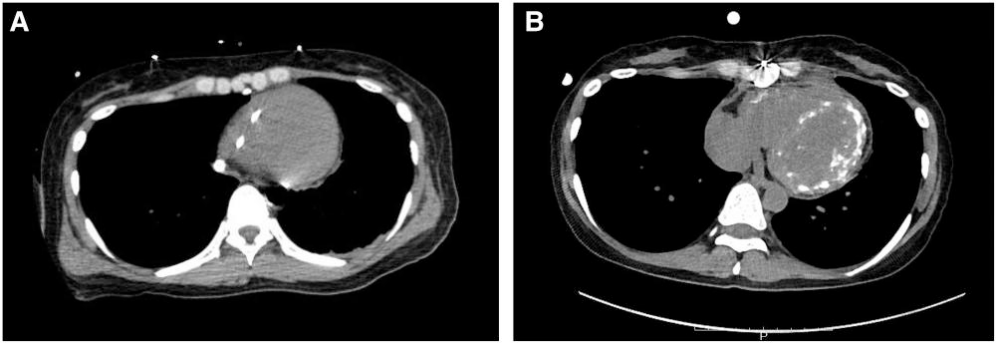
CT scan. (*A*) Before LVAD implantation. Swan-Gantz catheter in right ventricular. No apparent calcified myocardium. (*B*) Day 40 of admission. Extensive calcification in both ventricular myocardium.
